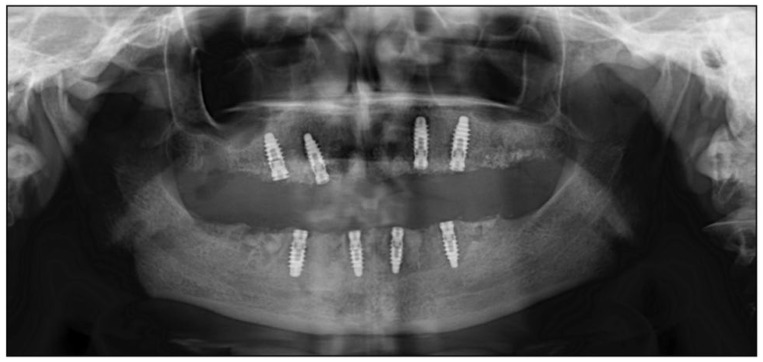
Postoperative (5 days after surgery) panoramic radiographs.
